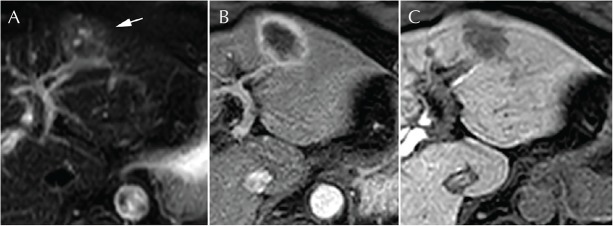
(A) The fat-saturated T_2_-weighted MR image reveals the shrunken nodule showing a dark signal (arrow) at follow-up examination after 21 months. (B) In addition, the arterial phase of the EOB-enhanced MR image reveals ring-enhancement, which is suspicious for its sclerosed change. (C) The hepatocyte phase of the EOB-enhanced MR demonstrates the hypointense-appearing nodule, but peritumoral hyperintensity is less prominent at this time.
